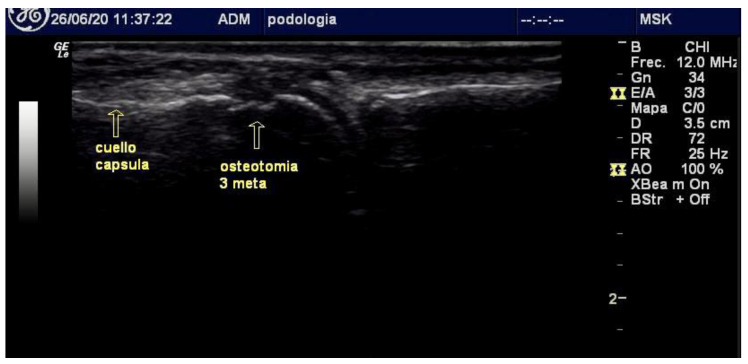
Ultrasound image showing how the intracapsular position of the osteotomy was evaluated. Considering that the joint capsule was inserted along the margins of the articular surfaces of the metatarsal head and the base of the corresponding phalanx, and that the articular cartilage is thicker on the plantar aspect of the metatarsal head, this enables accurate placement of the osteotomy within this region at the desired geometric location, under direct fluoroscopic and ultrasound guidance, using these anatomical landmarks.
